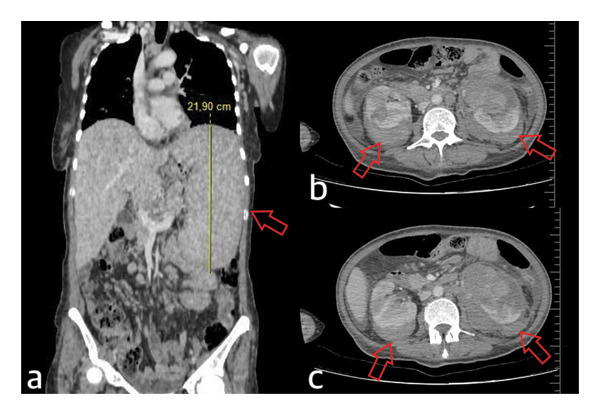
Contrast‐enhanced abdominal computed tomography (CT) images including portal venous phase acquisition. (a) Coronal portal venous phase image demonstrating marked splenomegaly (21.9 cm, arrow). (b‐c) Axial portal venous phase images showing bilateral perirenal and intrarenal hyperdense collections consistent with acute hemorrhage (arrows), without evidence of contrast‐enhancing renal mass, angiomyolipoma, aneurysm, or other detectable vascular malformation.
